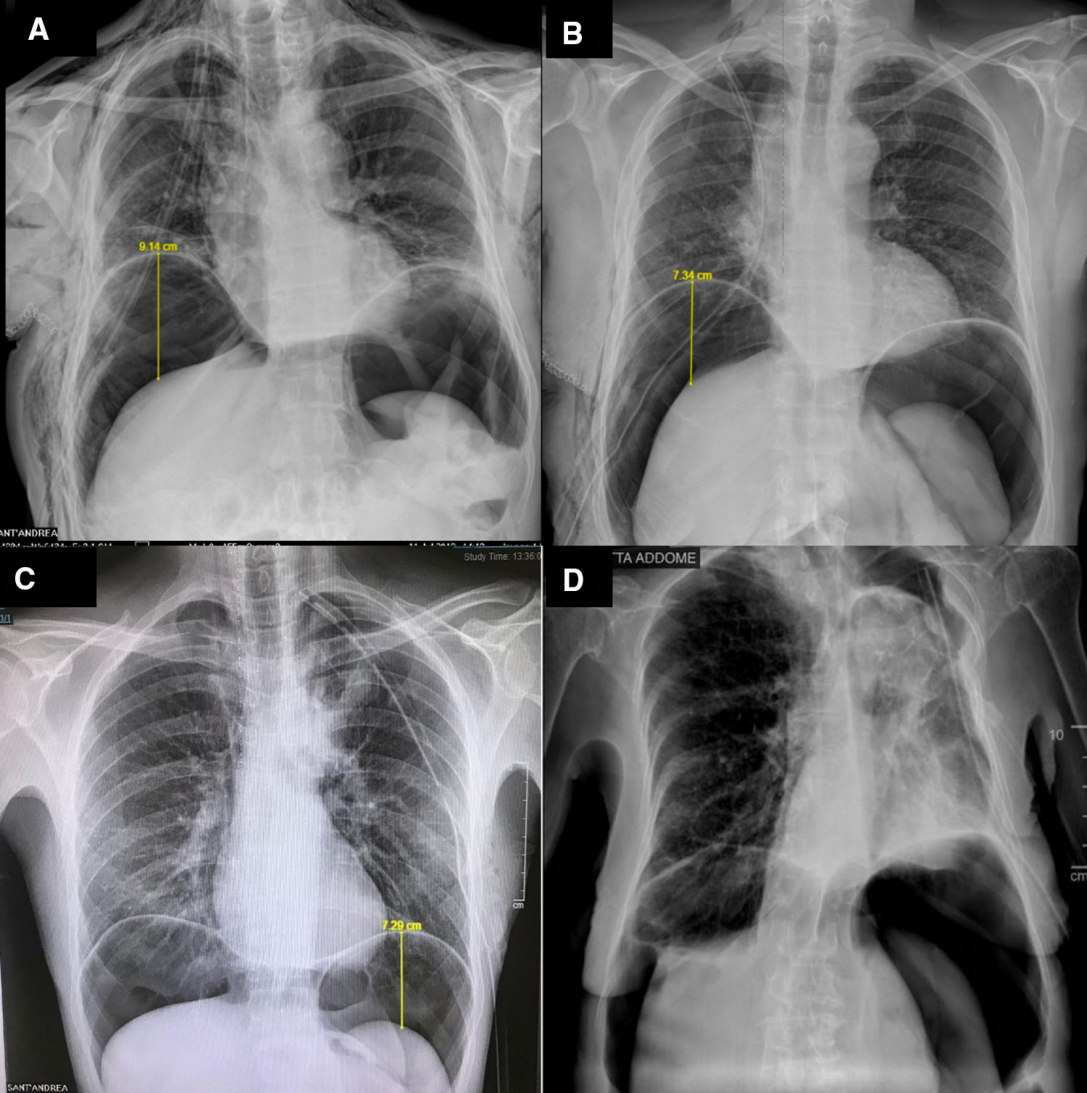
a, b Chest X-Ray 1 h after instillation of pneumoperitoneum for right pleural space; c, d chest X-Ray 1 h after instillation of pneumoperitoneum for left pleural space
